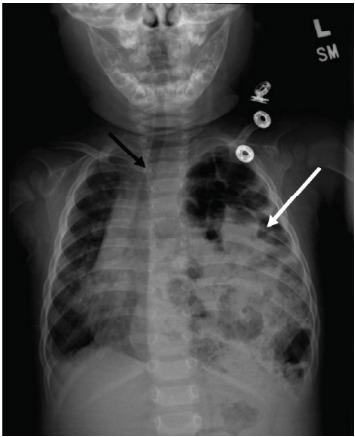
Chest radiograph with white arrow indicating an area of herniated bowel throughout the left hemithorax and black arrow indicating rightward tracheal deviation and mediastinal shift.
